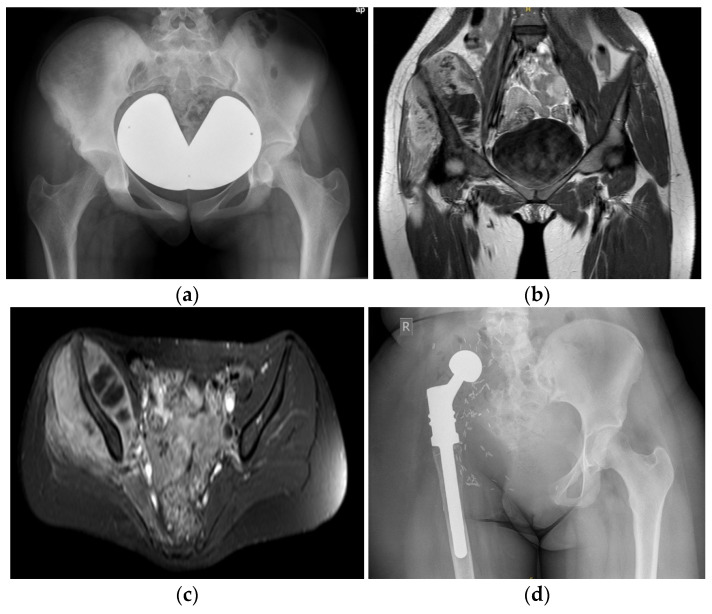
(a–d) Preoperative X-ray (a) of a 15-year-old male patient with an extensive high-grade osteosarcoma of the right ilium; preoperative MRI scans ((b), coronal view; (c), axial view); postoperative X-ray (d) after P1-4 hemipelvectomy and reconstruction with a modular endoprosthesis and attachment tube.
